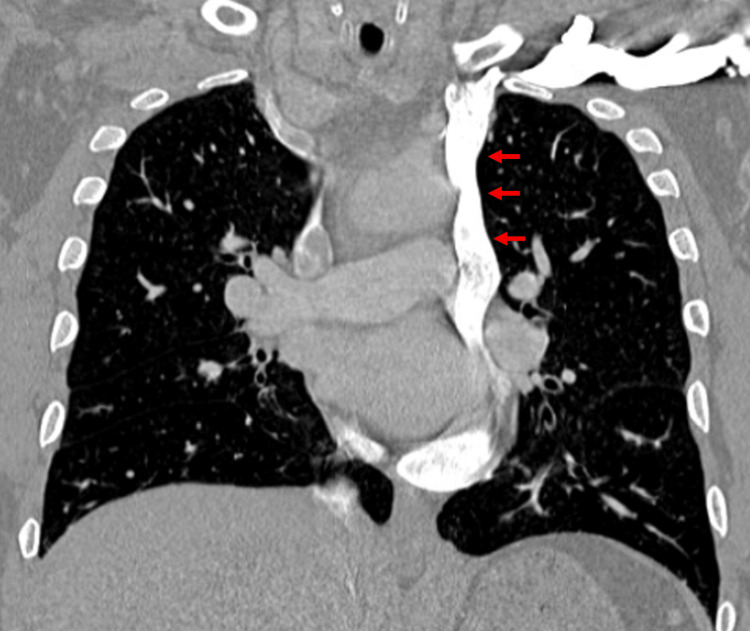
CT chest with contrast showing persistent left-sided superior vena cava (PLSVC) (red arrows) draining near the left heart or the coronary sinus
